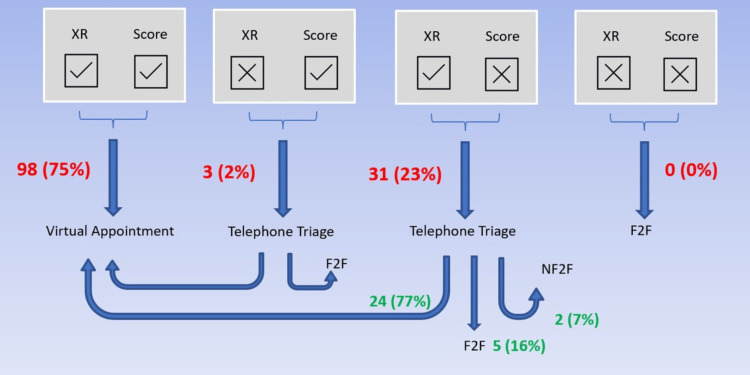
Number of patients in each domain at the one-year follow-up point. XR = X-ray; F2F = face-to-face; Nf2f = non-face-to-face
